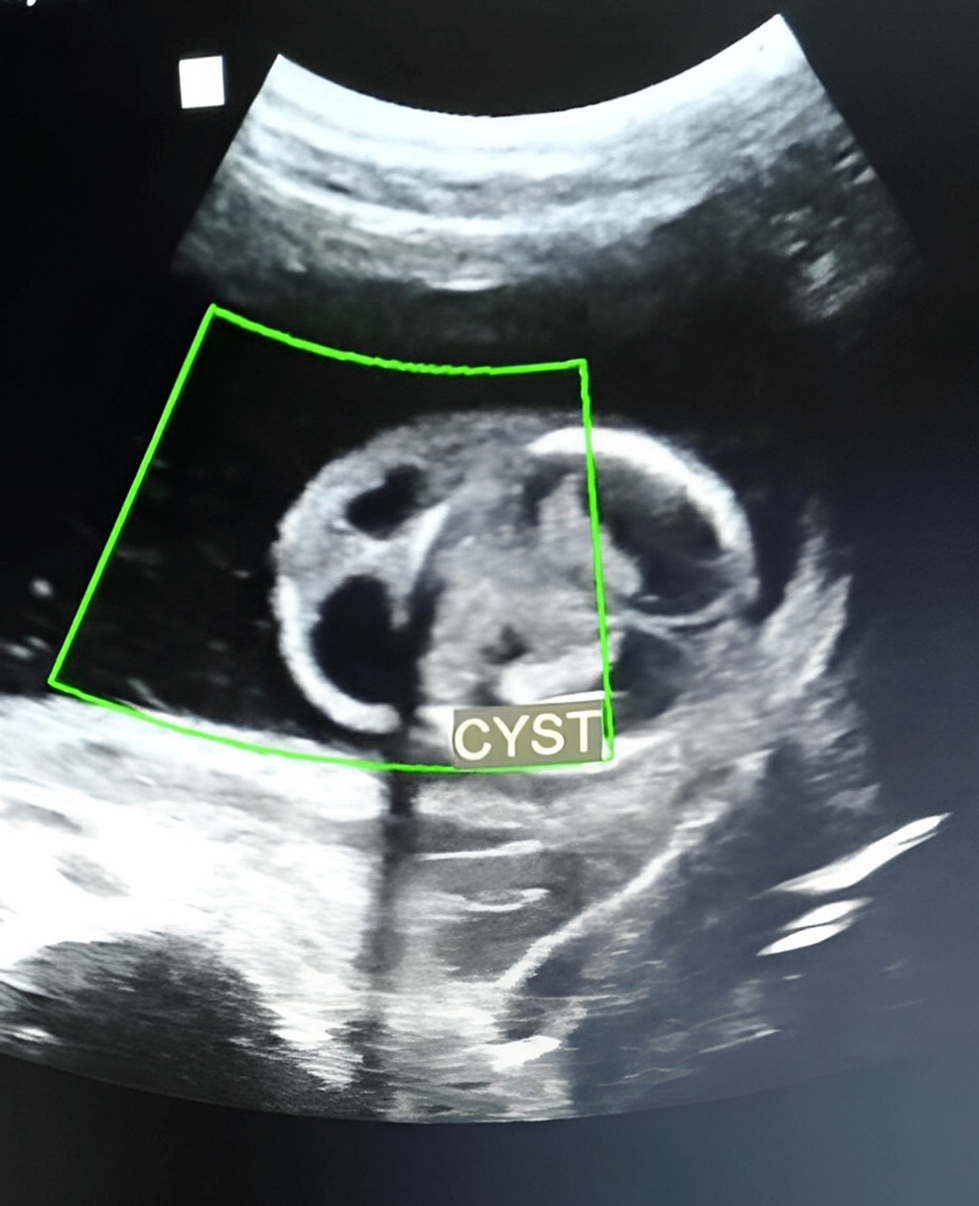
Color Doppler sonography does not show any flow in the lesion.
